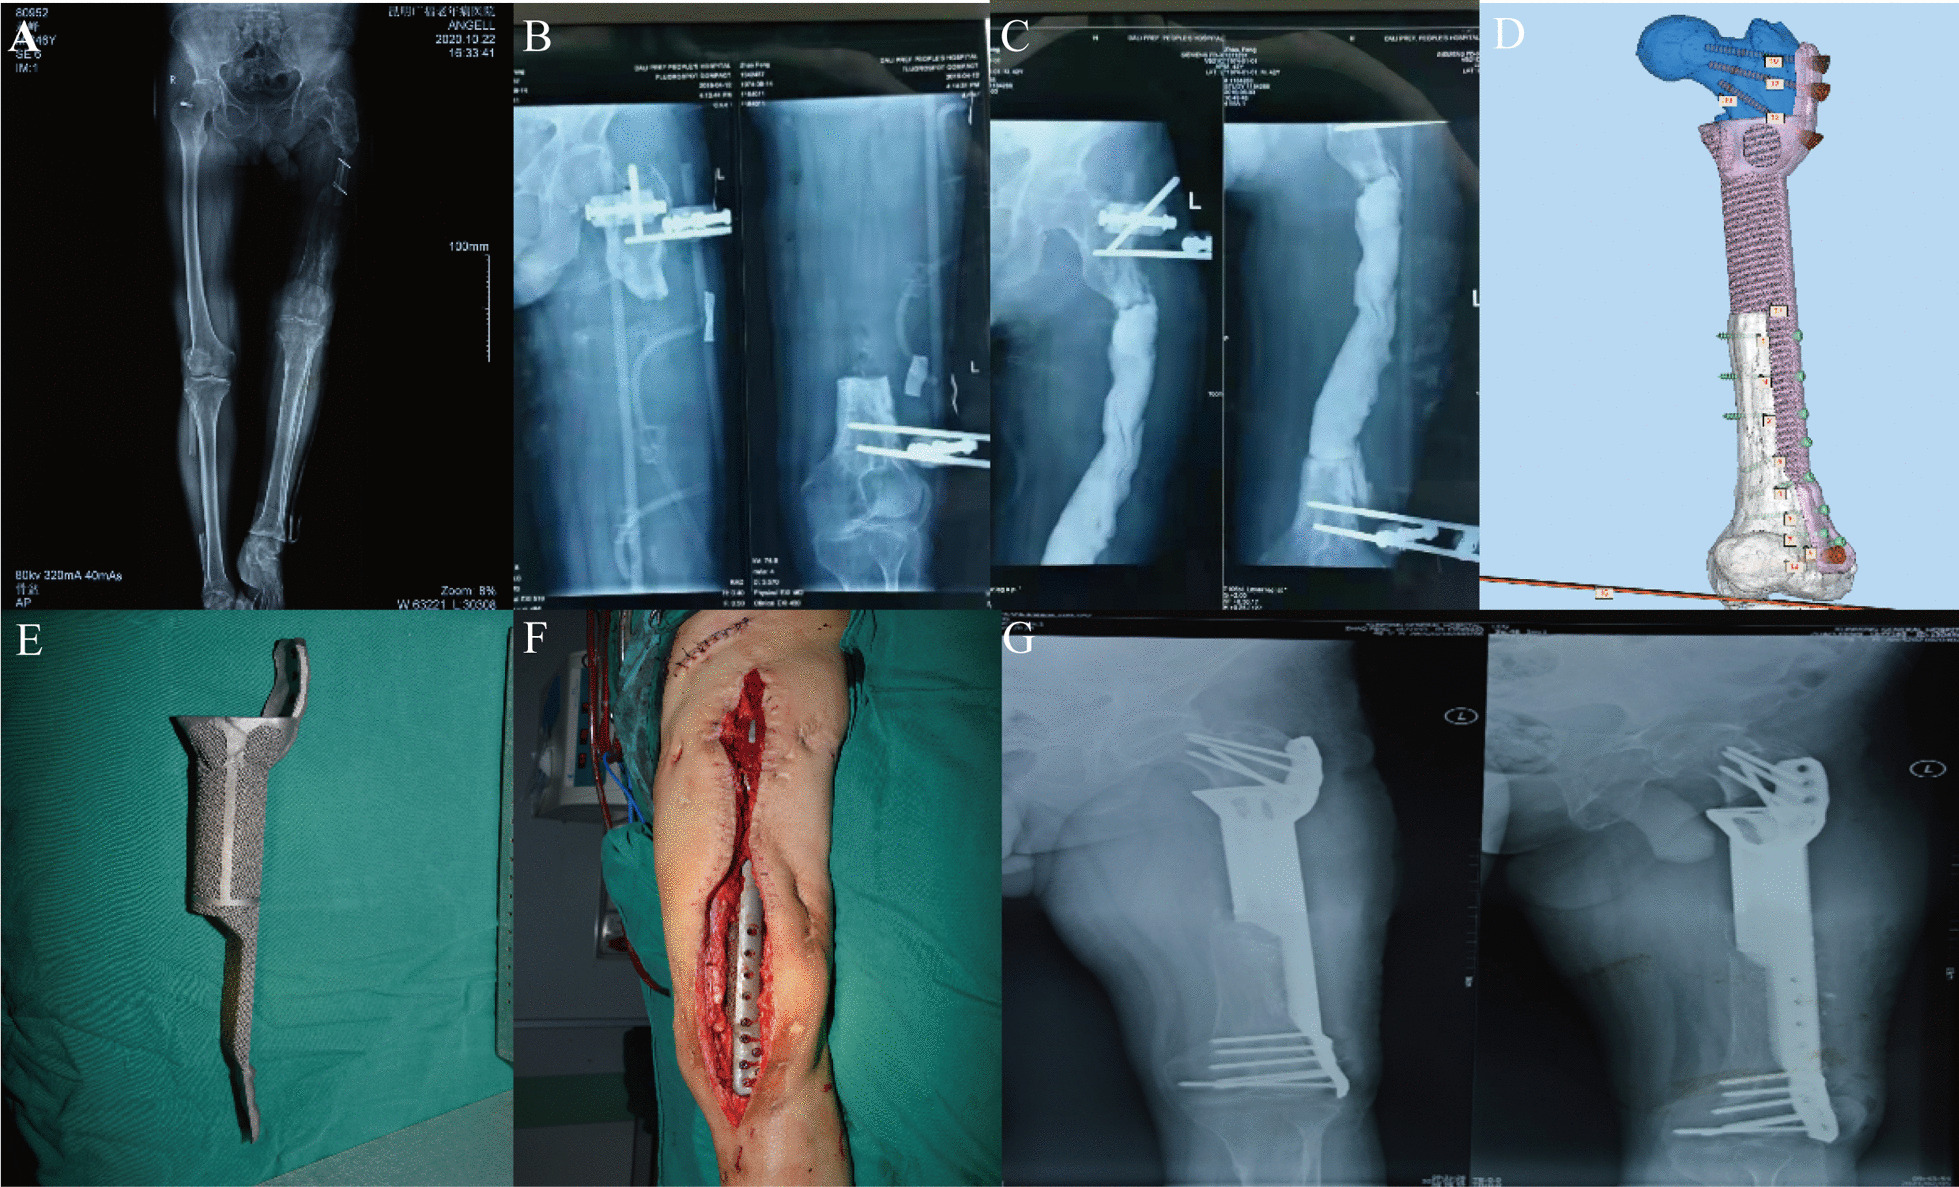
A A 60-year-old male with infective bone union on the left femoral, compared with the right-side limb, the patient presented serve varous deformity and length discrepancy. B The resection of infective necrosis bone was performed, used outfixation to sustain stability of the remained femur. C The vancomycin loaded bone cement spacer was filled in the bone defect section. D The design image of the customized 3D-printed prosthesis. E The photograph of real products, highly consistent with the specific shape of bone defect section. F Well fixation of the prosthesis after removing the outfixation and anti-infection filler. G X-ray image showed acceptable result without internal fixator failure in the last follow-up
